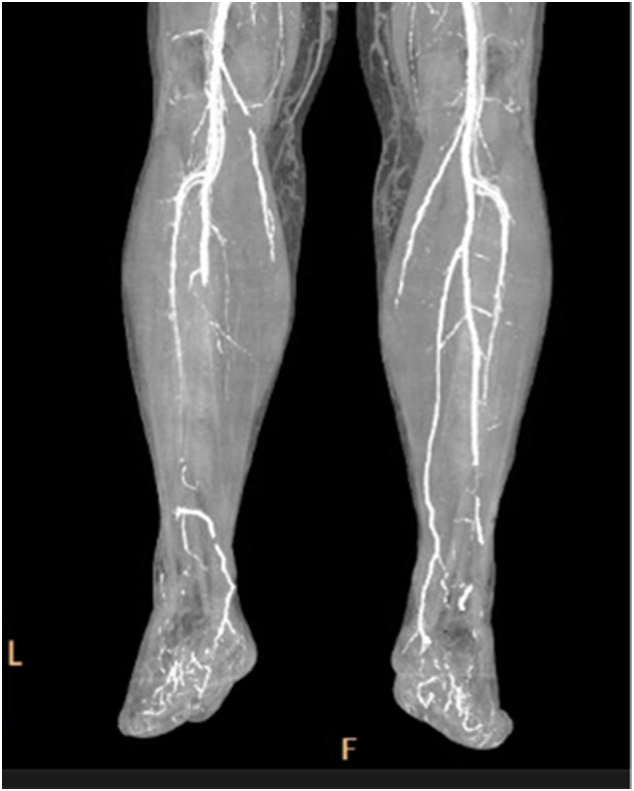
CT angiogram demonstrating ATA/PTA occlusion and Peroneal Artery stenosis.
